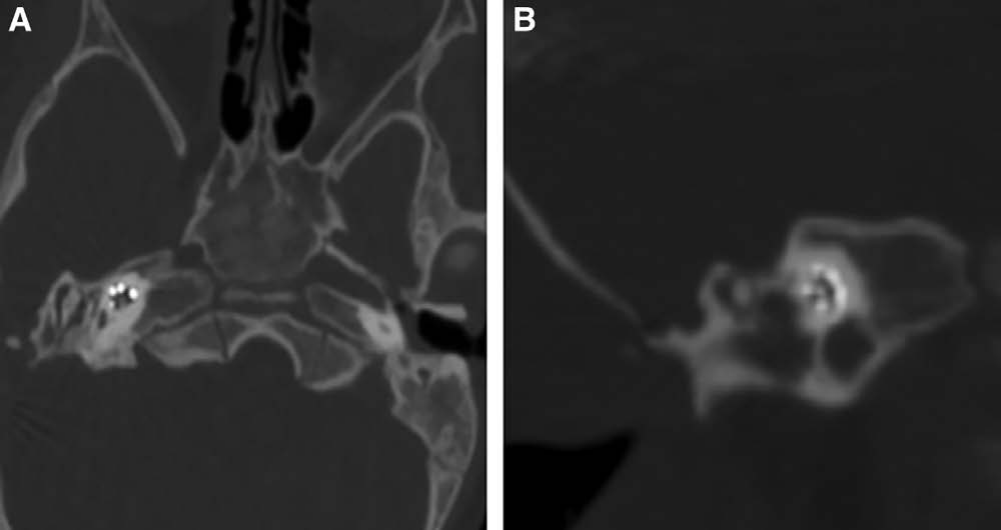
Postoperative CT of the patient. Axial (A) and coronal CT (B) showed all the electrodes were inserted into the cochlea (right side) and there was no folded or dislocation of the electrodes. CT = computed tomography.
